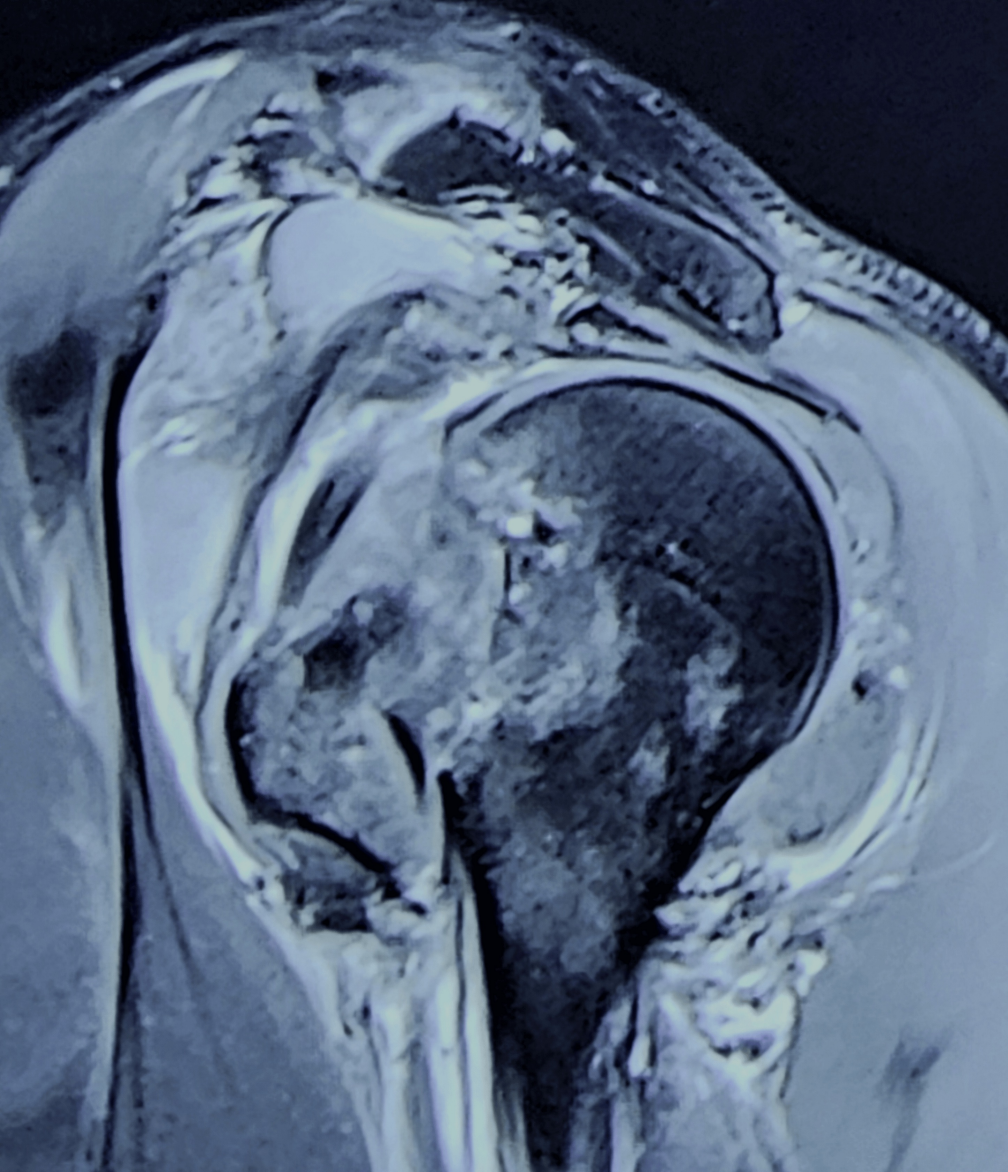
MRI (sagittal view) showing GT fracture with a subluxated long head of biceps tendon from the bicipital groove. MRI, magnetic resonance imaging; GT, greater tuberosity
